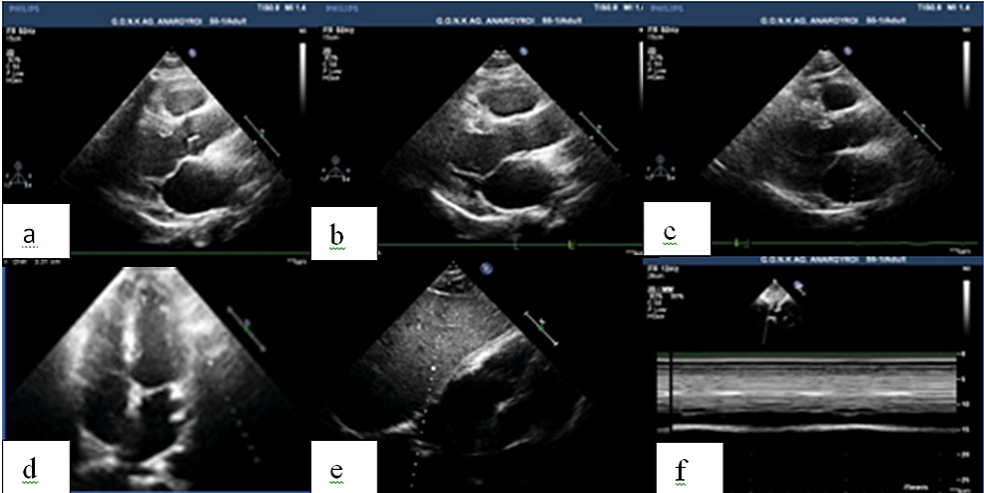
Cardiac ultrasound. Parasternal long axis view (a,b,c), apical 4 view (d), and subcostal view (e,f).
